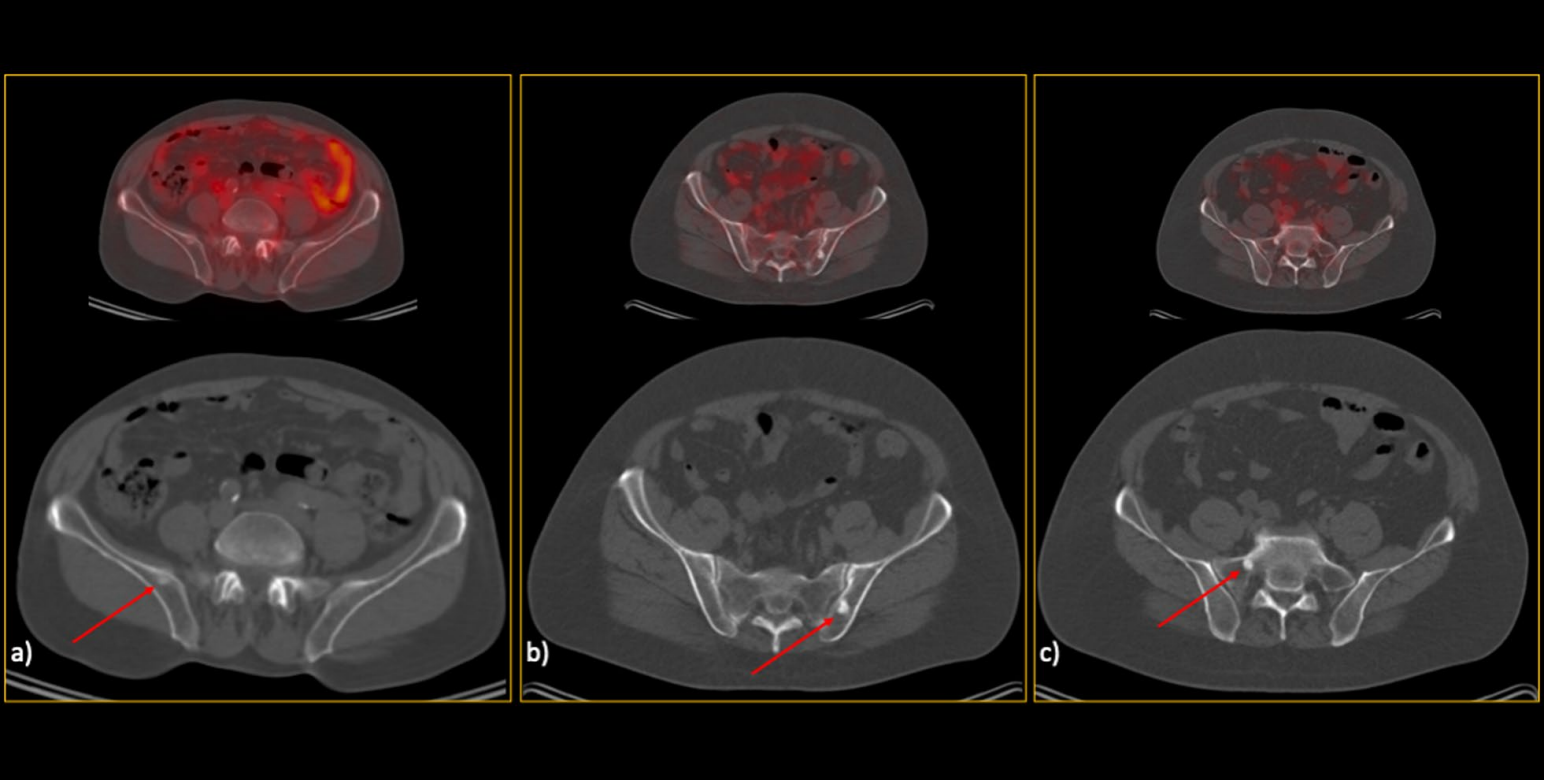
False-positive low-dose CT findings (red arrows). The majority of the false-positive findings were located in the pelvic region, most likely due to the readers’ knowledge about the high pretest probability in this region. So, as can be seen (a–c), there were various lesions called metastatic on CT images but were found benign considering the reference standard. Noteworthy, although b and c might seem not that much challenging and in favour of bone islands while looking at only one slice, in patients with multiple metastases, they were falsely interpreted as malignant since there were also other lesions with more or less similar Hounsfield unit in the same patient
